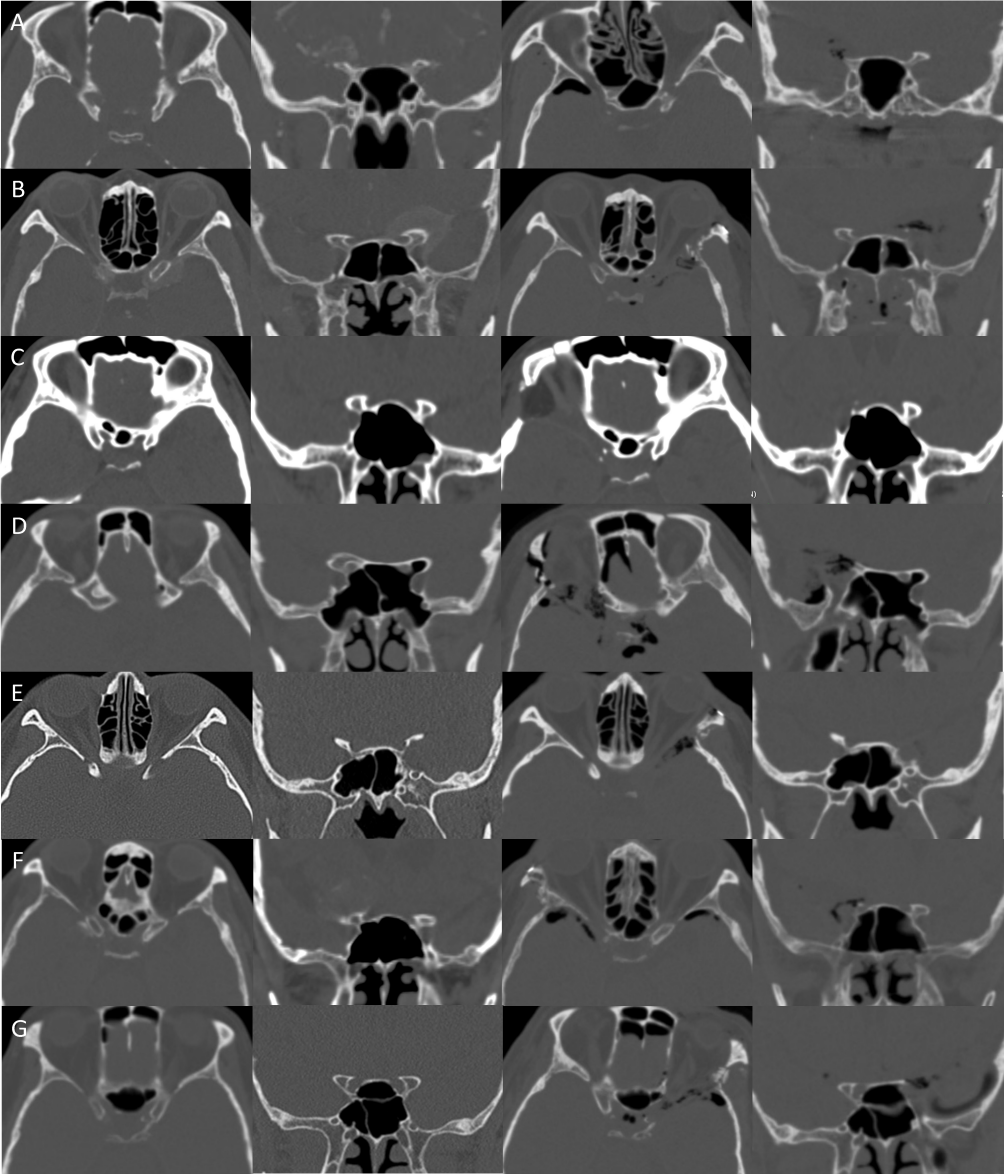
Clinical implication. (A–G) In seven cases, the stepwise extradural anterior clinoidectomy was completed. Complete removal of the ACP was confirmed with postoperative CT. CT, computed tomography.
